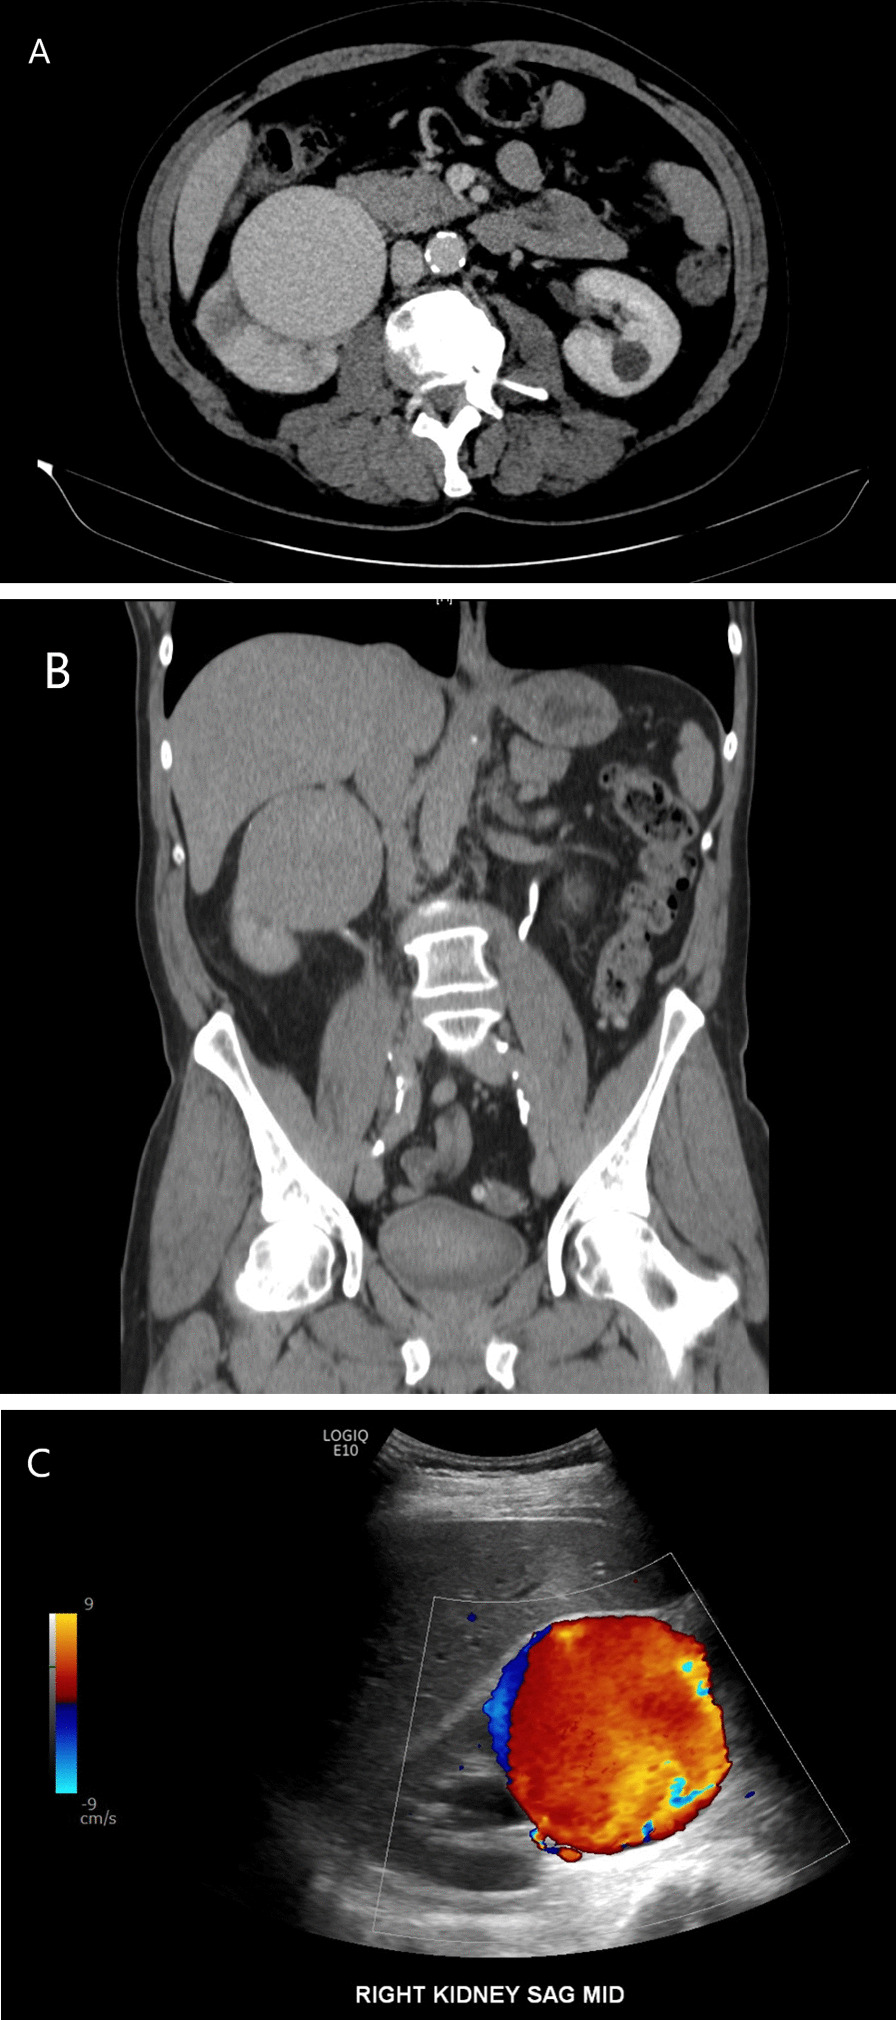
CT with intravenous contrast (a, b) demonstrated a 7.5 × 7.2 cm ovoid mass that was compressing on the upper pole and interpolar regions of the right kidney. The mass had similar density to blood. Doppler ultrasound (c) showed pulsatile flow within the right renal mass
